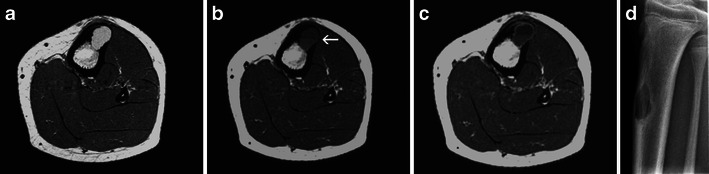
Non-ossifying fibroma—incidental finding on MRI: The lesion is hyperintense on T2 (a), hypointense on T1 with a fluid-fluid level (*arrow*; b), and it presents peripheral contrast enhancement (c). Lateral X-ray of the tibia demonstrates the typical NOF aspect (d)
